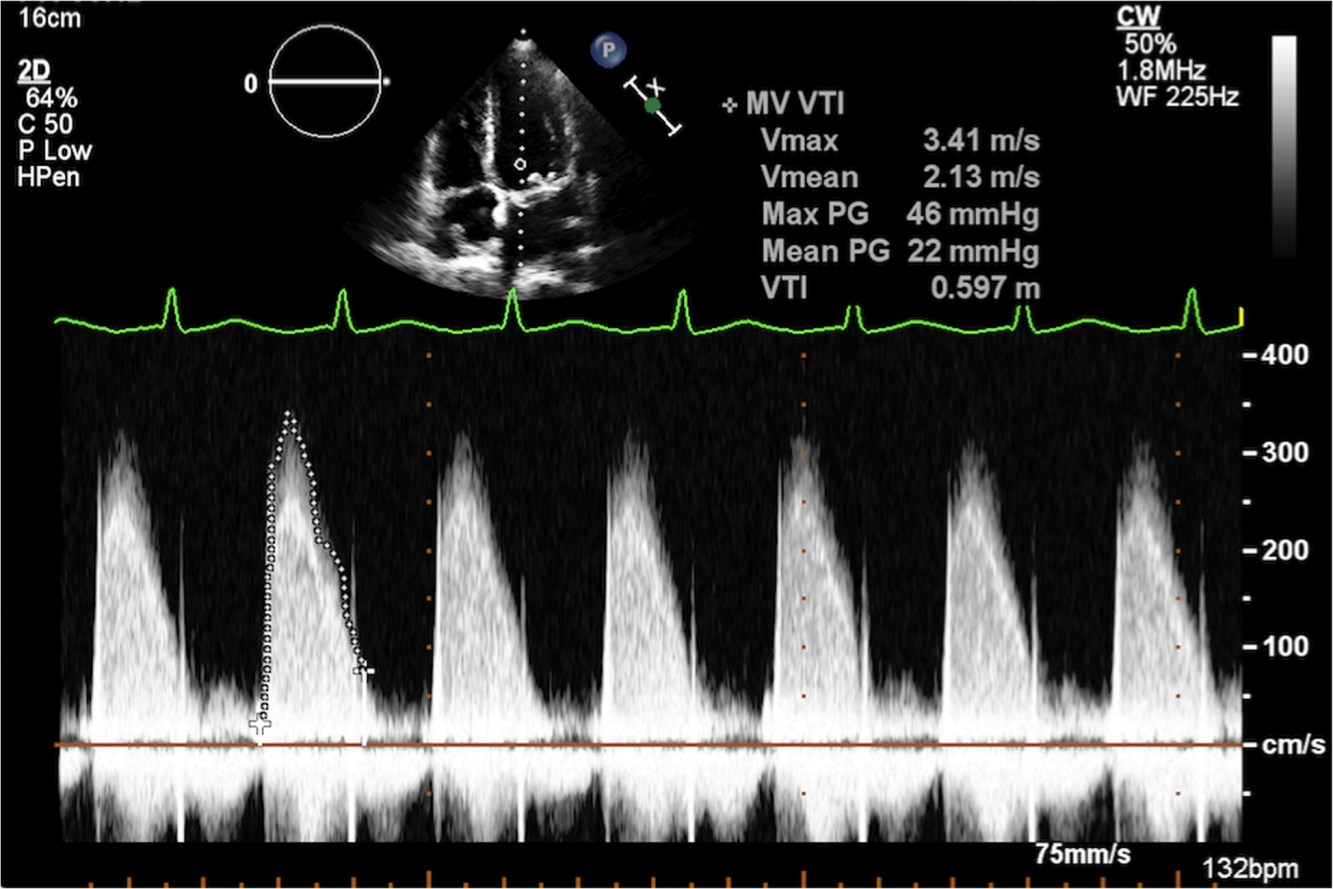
Doppler imaging by transthoracic echocardiography demonstrating a marked elevation in mean transmitral diastolic gradient of 22 mmHg at a heart rate of 132 beats per minute, indicating significant stenosis across the mitral prosthesis
